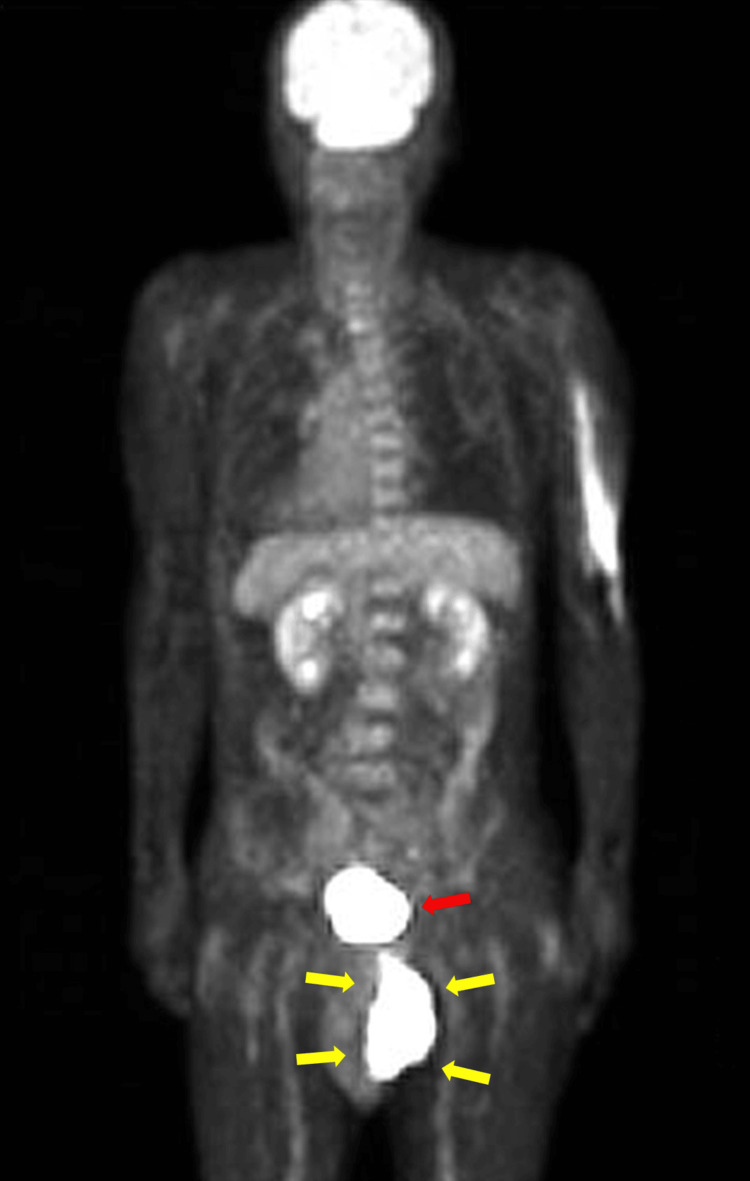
FDG-PET/CT scan of Case 1 (posterior-to-anterior view) Fluorodeoxyglucose positron emission tomography/computed tomography (FDG-PET/CT) scans showing a tumor in the anal canal (yellow arrows). Because the scan was performed in the prone position due to difficulty lying supine (the mass was protruding externally from the anus), the image orientation is posterior-to-anterior, resulting in a reversed appearance of the heart and other thoracic structures. The focal uptake in the right upper arm corresponds to extravasation of the radiotracer during injection. Physiological tracer accumulation in the urinary bladder is also visible (red arrow), located superior to the anal canal tumor.
